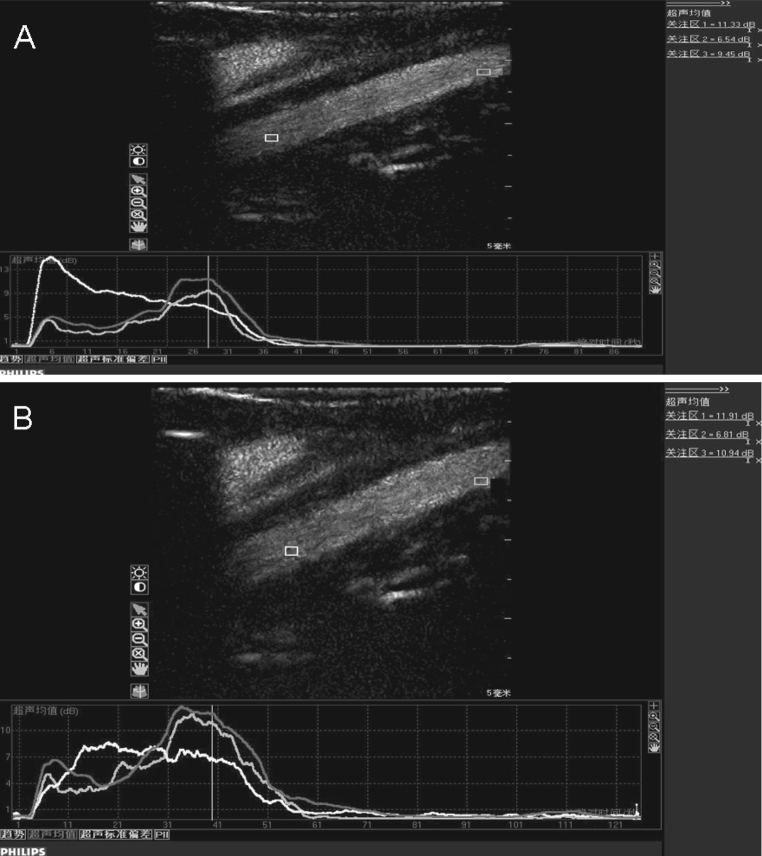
The time-intensity curves of contrast ultrasound imaging with normal microbubbles (a) and IL-8-targeted microbubbles (b). The *yellow*, *blue* and *red* curves indicate the intensity of the lumen, intima and plaques, respectively. (Color figure online)
